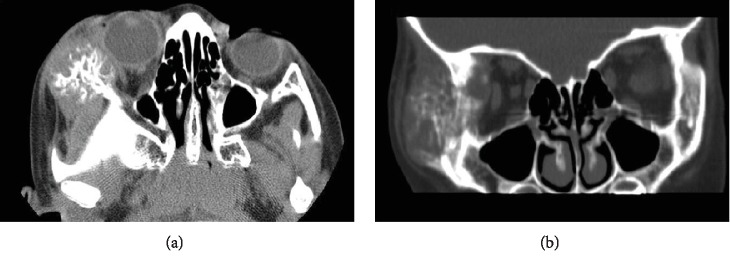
(a) Axial and (b) coronal noncontrast computed tomography (CT) of the orbit of an osteolytic lesion with a large soft tissue component involving the right frontal and lateral orbit and zygomatic arch. A sunburst- or honeycomb-type pattern of new bone formation is seen.
